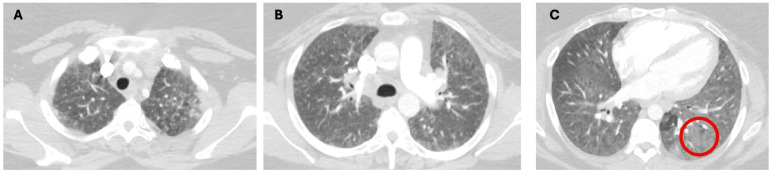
Chest CT images of a patient with inflammation-predominant ILD showing ground-glass opacities (image (C)). Image (A–C) show cross-sectional views of chest CT images of a patient with inflammation-predominant ILD. Red circle shows an area of ground-glass opacities.
